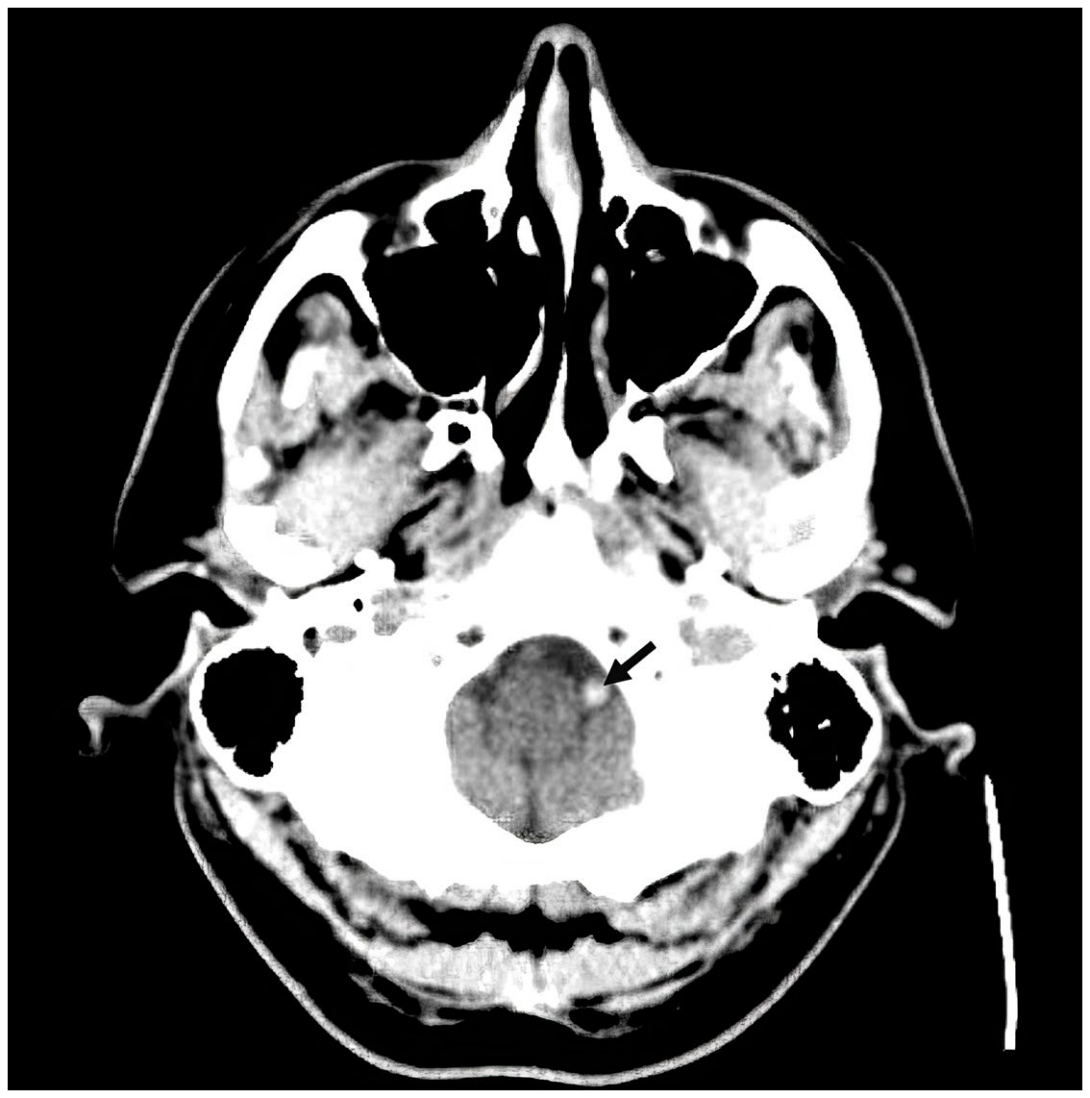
Non-contrast CT head demonstrating hyperdense vessel sign in the left intracranial vertebral artery (arrow).
